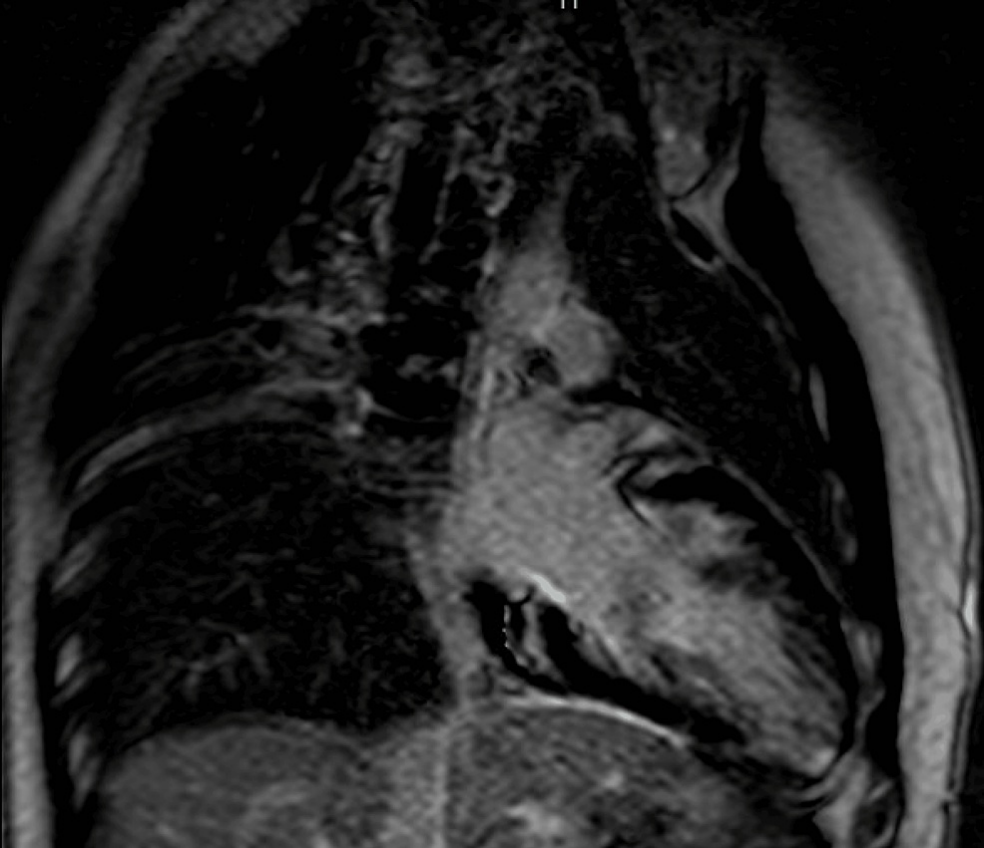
Cardiac MRI The ratio of non-compacted to compacted myocardium is more than 4:1, indicating LVNC cardiomyopathy, with non-compacted myocardium more prominent in the apex. LV: left ventricle; LVNC: left ventricle non-compaction
